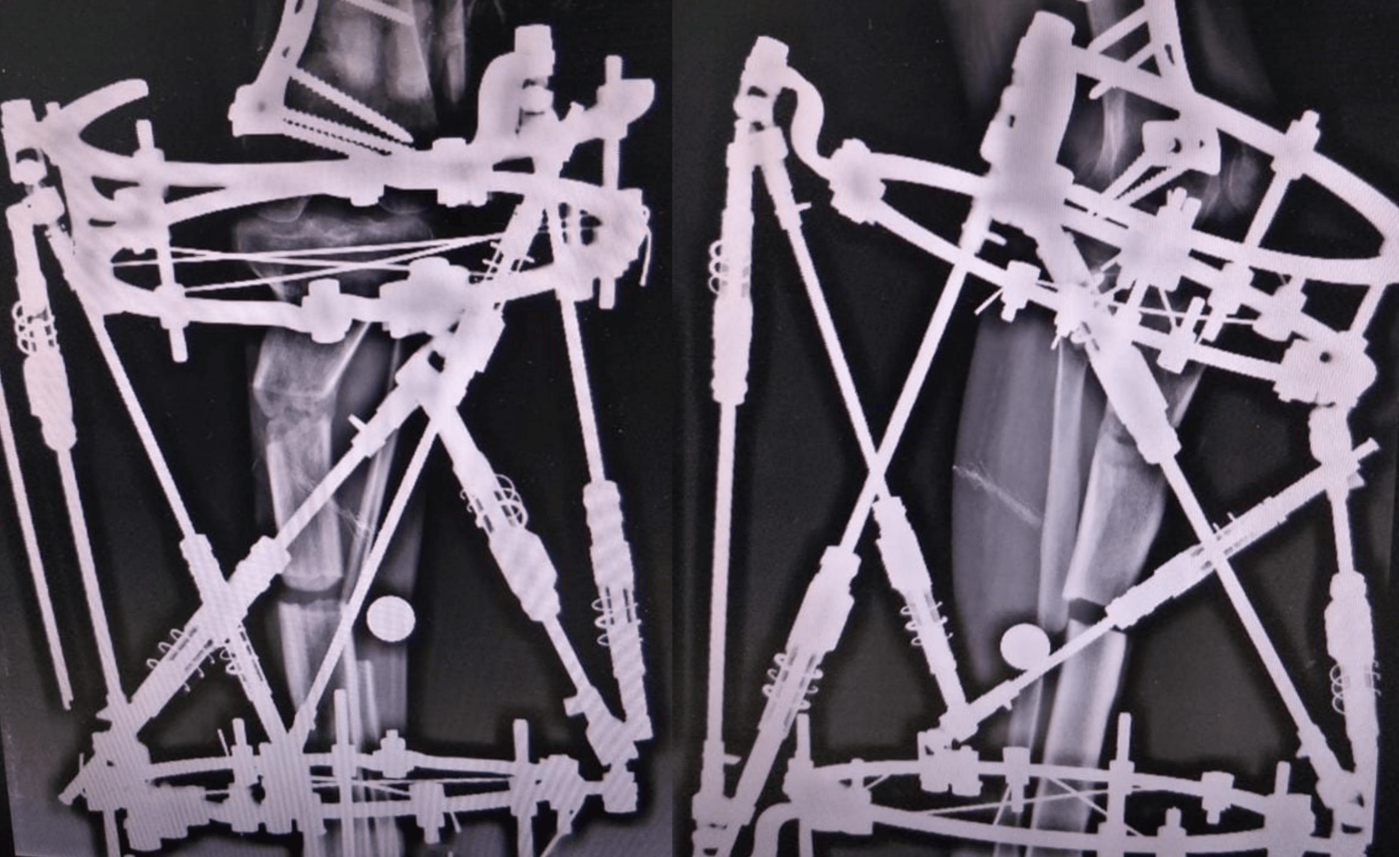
The anteroposterior and lateral views of the deformity correction using the Ortho-SUV fixator.
